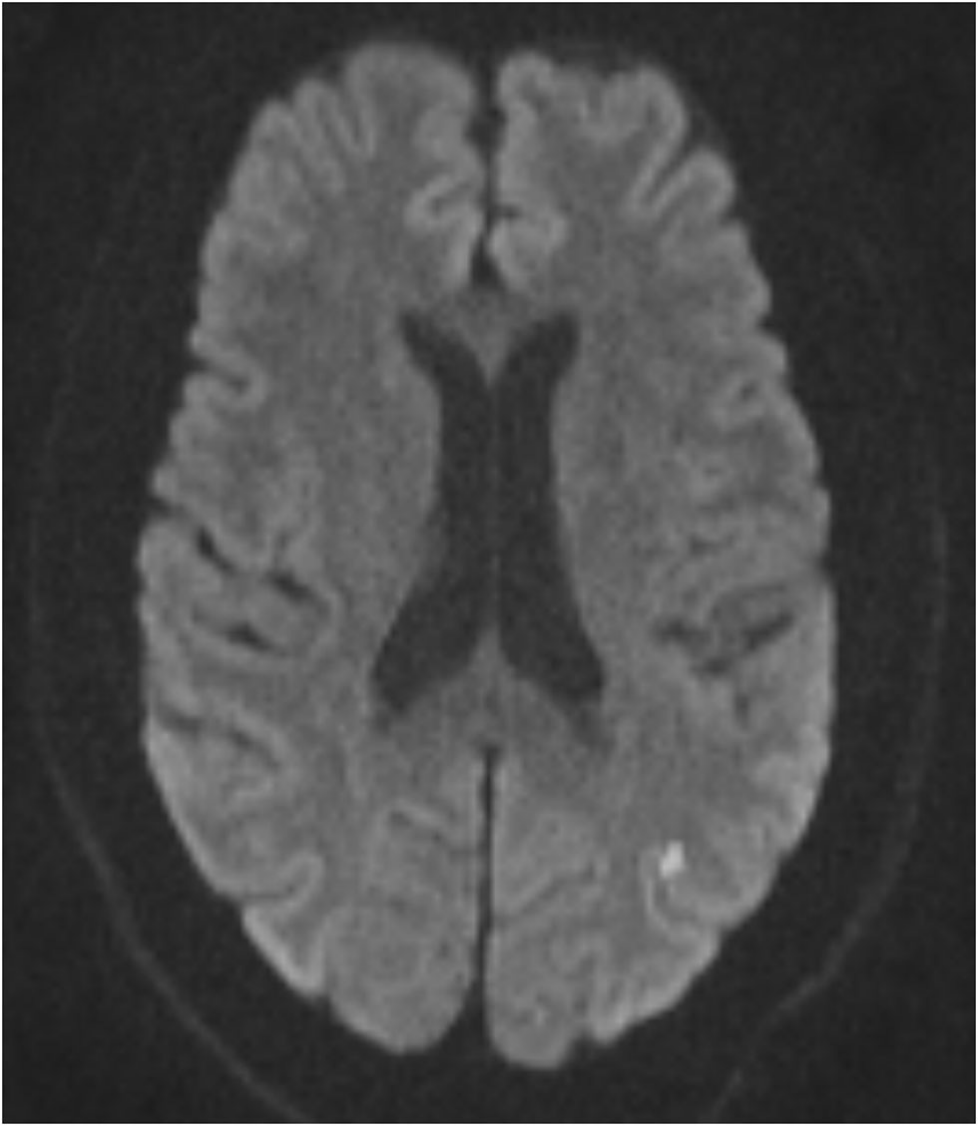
MRI DWI sequence of Cortical/Territorial Infarct.
